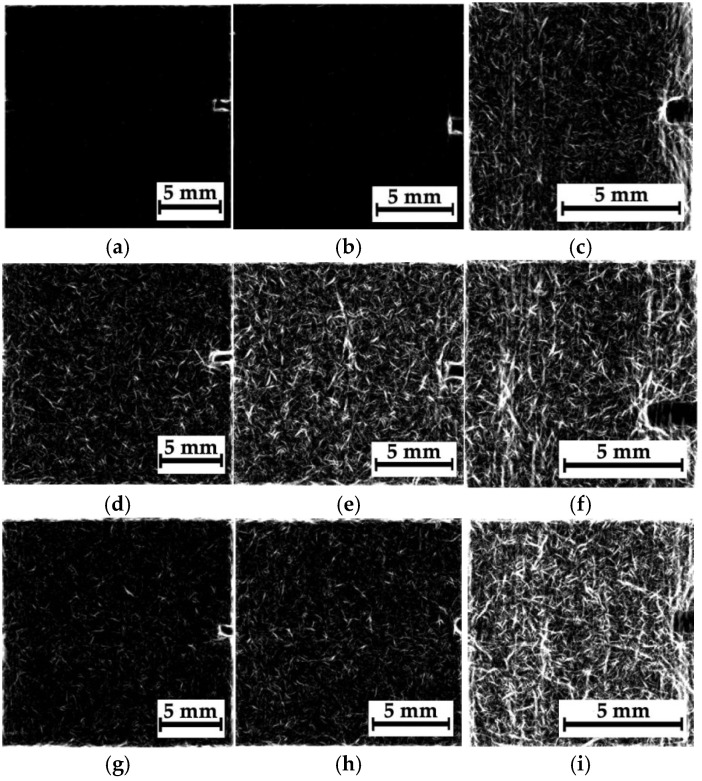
The phase variations of the radiographic images shown in Figure 8a–c; the distances between the sample and the sensor are 39 mm (a,d,g), 160 mm (b,e,h), and 240 mm (c,f,i).
